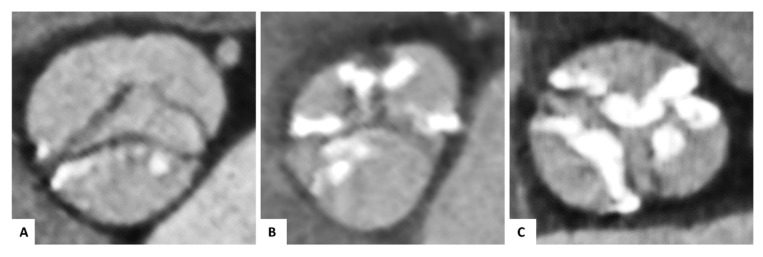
Semiquantitative calcification assessment of the device-landing zone. Pre-TAVI contrast-enhanced CTA of congenital and functional bicuspid aortic valves for visual calcification assessment with examples for mild (A), moderate (B), or severe (C) calcification of the cusps regions.
